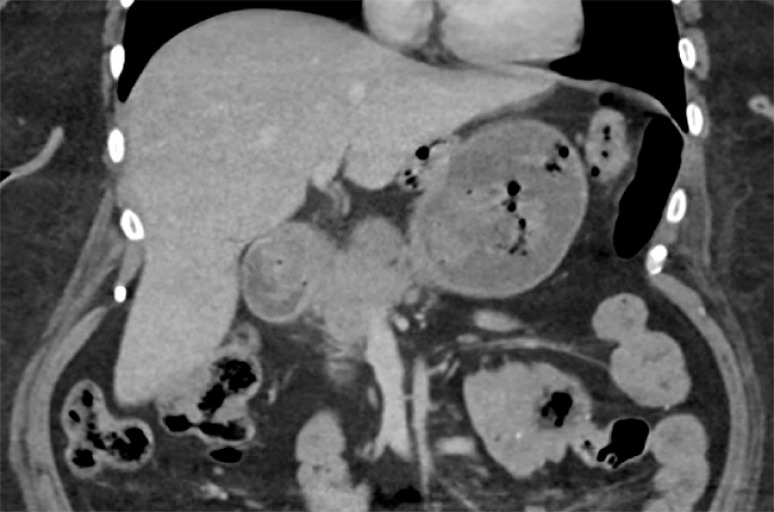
Computed tomography demonstrating high-density fluid in the descending duodenum, suggestive of hemorrhagic fluid.
